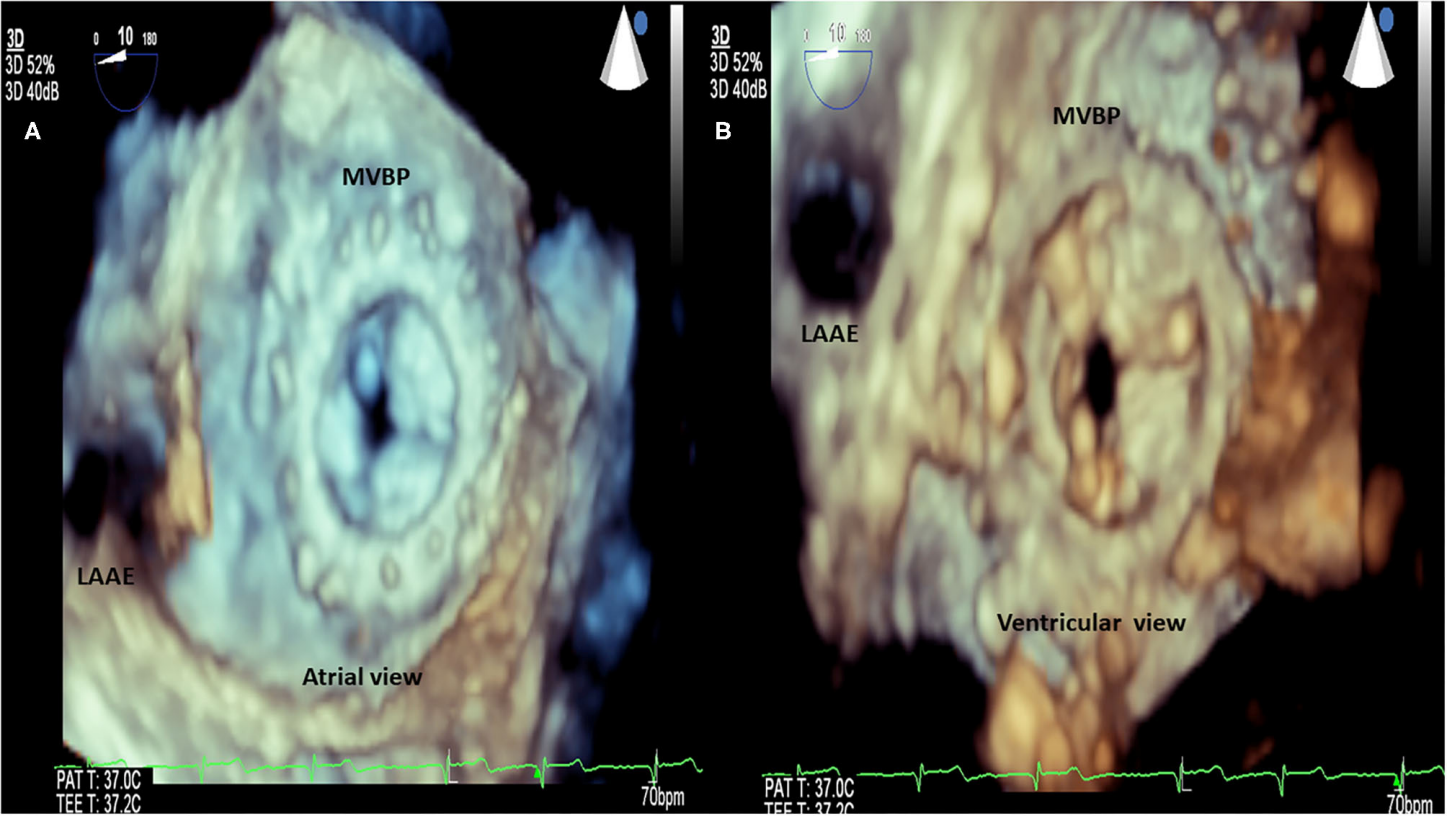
RHD (mitral valve stenosis, stenotic bioprosthesis). 3D transesophageal echocardiography. Demonstration of mitral valve bioprosthesis stenosis. (A) Atrial view. (B) Ventricular view. LAAE, left atrium appendage; MVBP, mitral valve bioprosthesis.
